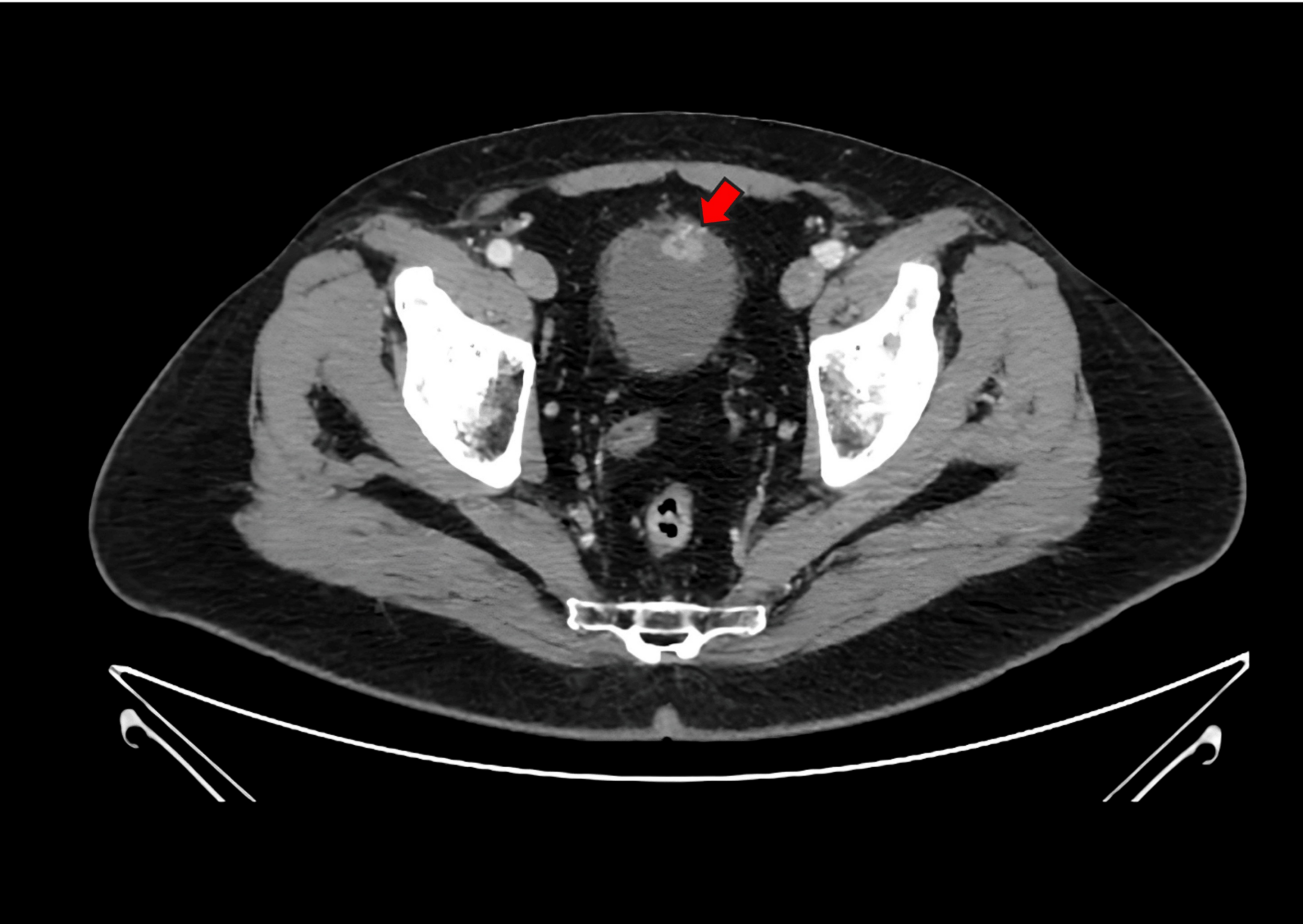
Contrast axial abdomenipelvic CT scan demonstrating an enhancing mural nodule on the anterior bladder wall suggestive of malignancy. The red arrow in the image points to an enhancing mural nodule on the anterior bladder wall measuring 1.0 × 1.7 × 1.0 cm, with prominent feeding vessels arising from the left inferior vesical artery.
